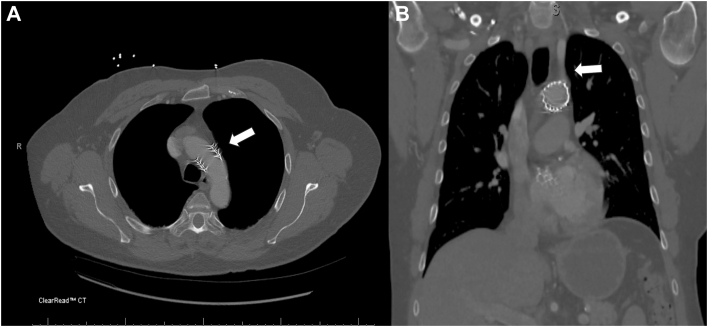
(A) Postprocedural computed tomography. The embolized valve was located at the aortic arch near the origin of the left subclavian artery (white arrow). (B): Patent left subclavian artery (white arrow).
